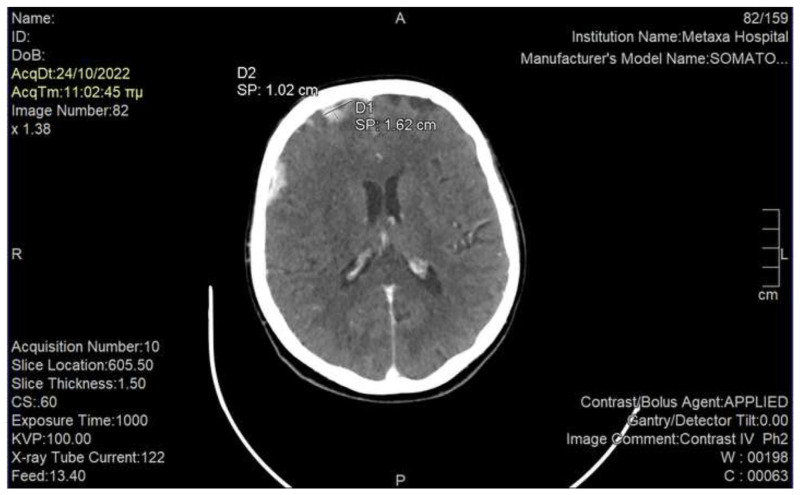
Brain CT showing a 1.6 × 1 cm metastatic brain lesion in the right orbitofrontal cortex, which was considered to cause the patient’s phantosmia.
